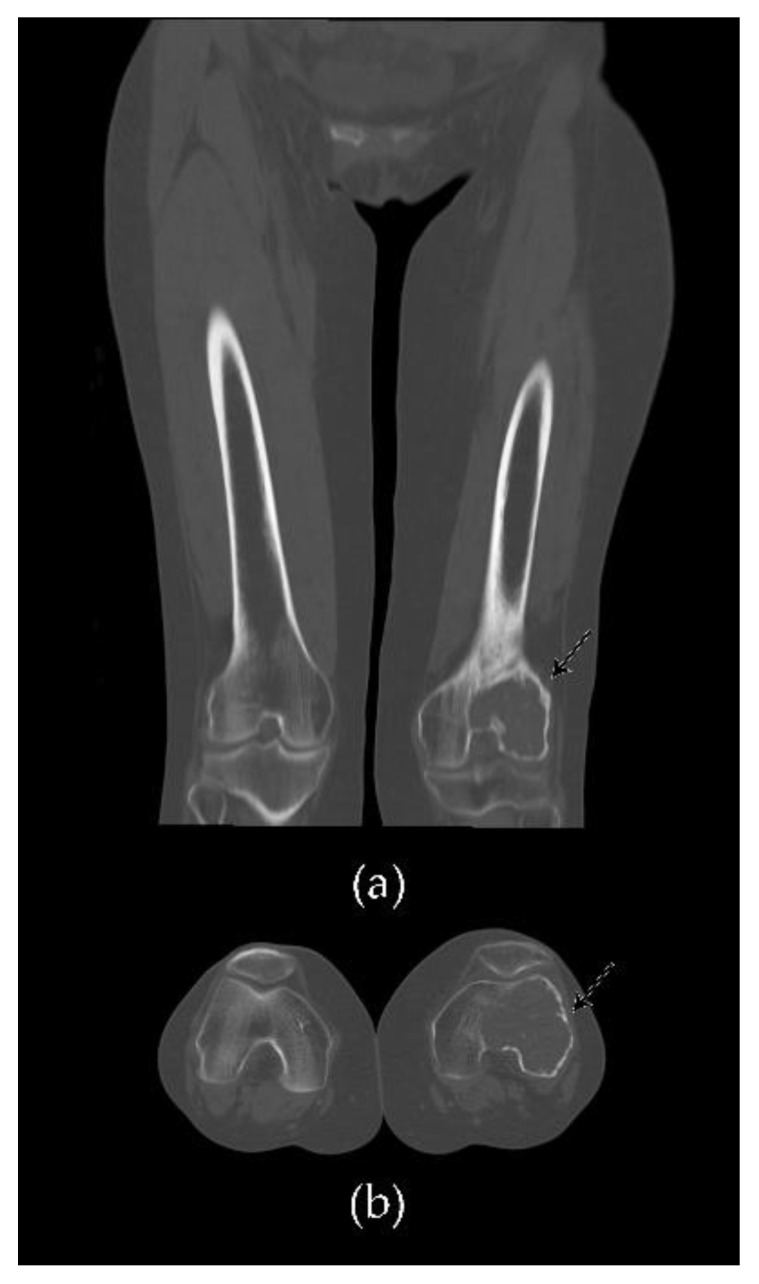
Comparative CT scan of a case of GCTB of the lateral condyle of the left femur: (a) coronal and (b) axial views demonstrate an extensive osteolytic lesion with cortical thinning (arrows). Note the osteo-structural alteration with a loss of bone trabeculation in comparison with the contralateral right normal limb.
